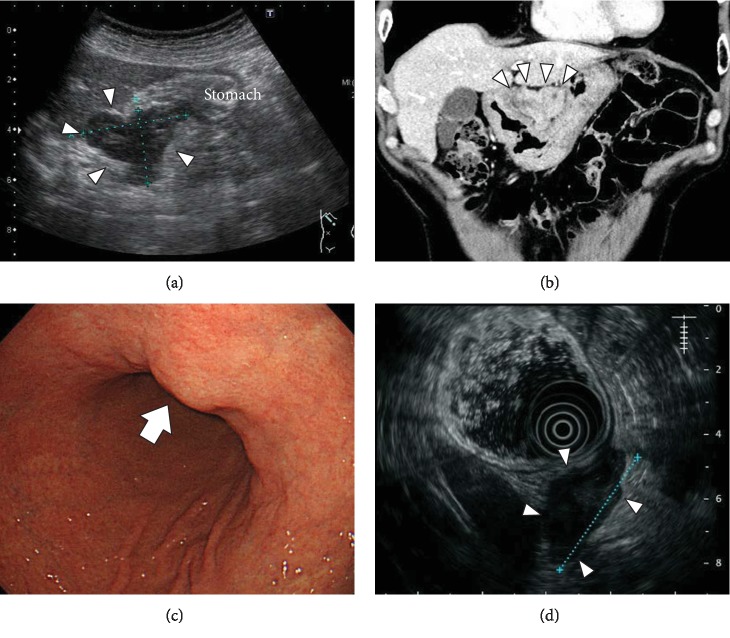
Preoperative imaging findings. Abdominal ultrasonography (AUS) showing a leaf-shaped tumor (arrowhead) with a mosaic pattern. (a) Computed tomography (CT) showing internal heterogeneity (arrowhead) between the head portion of the pancreas and the left liver lobe, (b) upper gastrointestinal endoscopy showing an elevated, nonepithelial lesion with a smooth surface in the lesser curvature of the stomach (arrow), (c) endoscopic ultrasonography (EUS) showing the same tumor (arrowhead) that seemed to be continuous with the fourth layer, and (d) the tumor was approximately 49 × 29 mm^2^ in size. The tumor mass appeared to protrude from the stomach wall.
